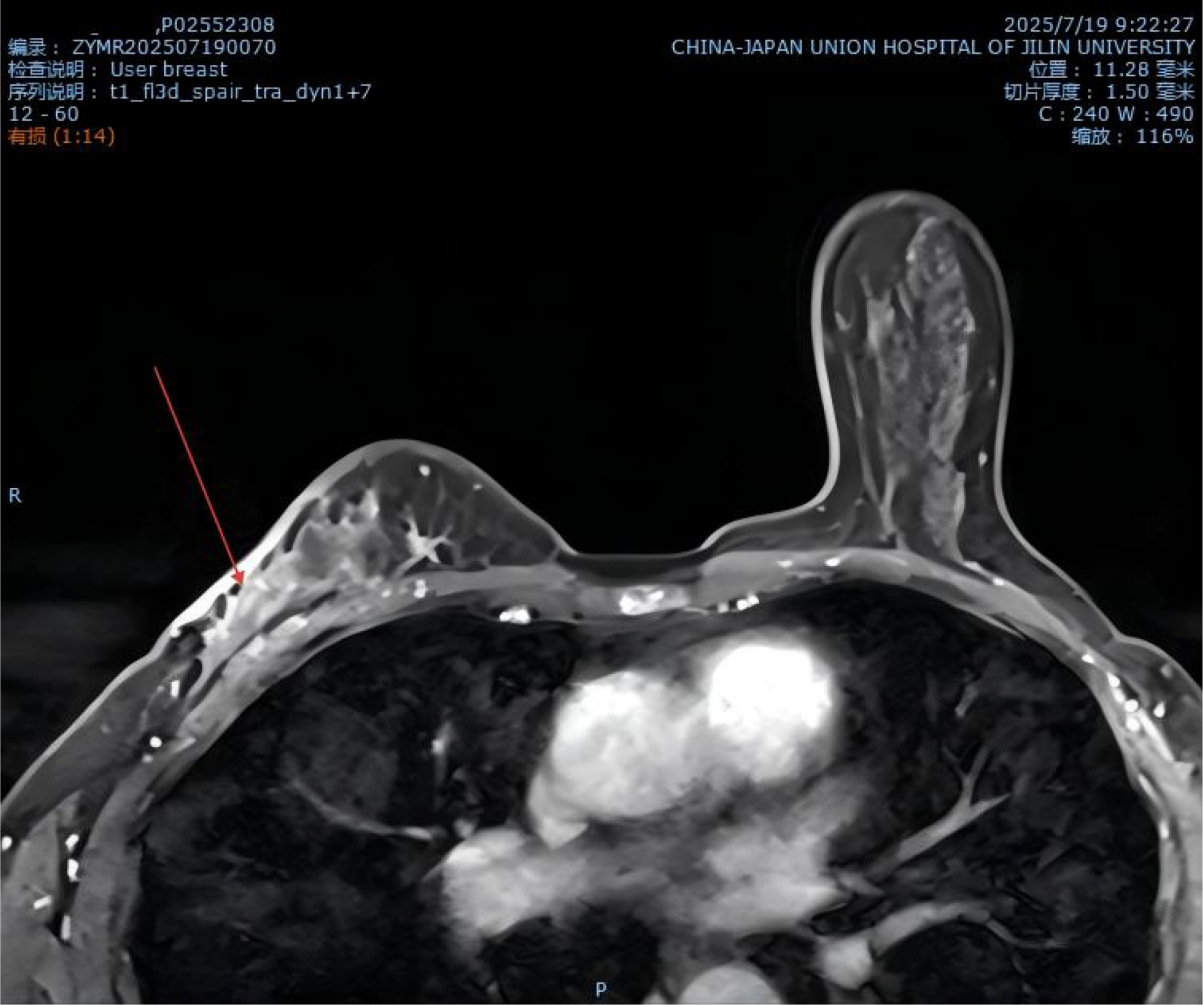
MR image from July 2025.
